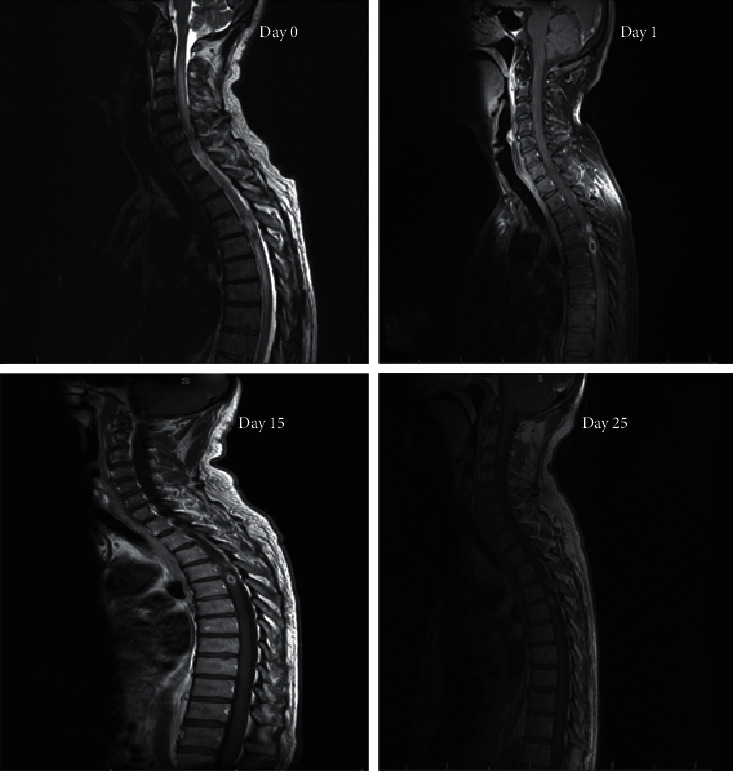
MRI of the spine with gadolinium contrast performed at days 0, 1, 15, and 25. Initial examination revealed a small cavitating mass (7.3 mm) within the left thoracic hemicord at the level of T5 and nodule (4 mm) at T4 with associated marked cord oedema and meningeal thickening which peripherally enhanced on contrast administration. By day 25, the lesion had shrunk to 5.5 mm with marked improvement in cord oedema and meningeal thickening. The superior T4 nodule remained unchanged in appearance.
